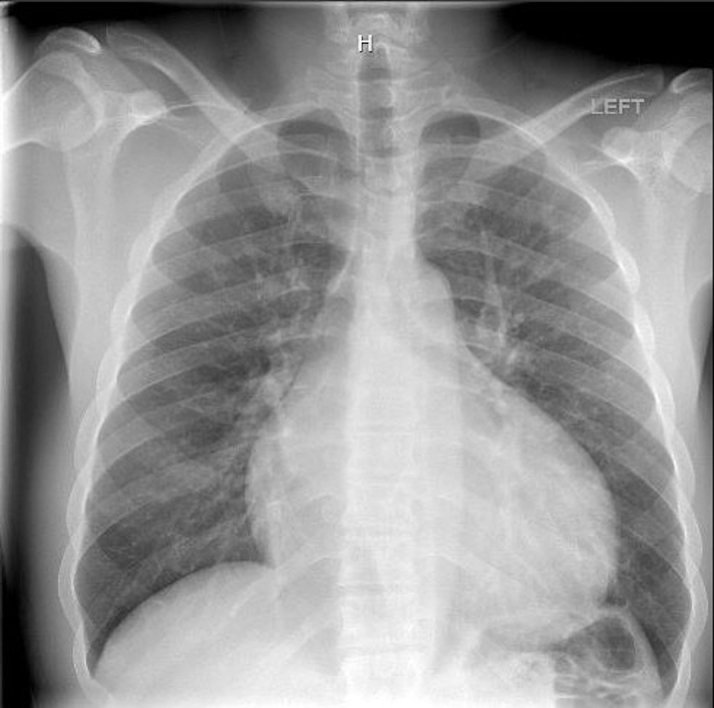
Chest X-Ray Demonstrating Cardiomegaly and Pulmonary Vascular Congestion
